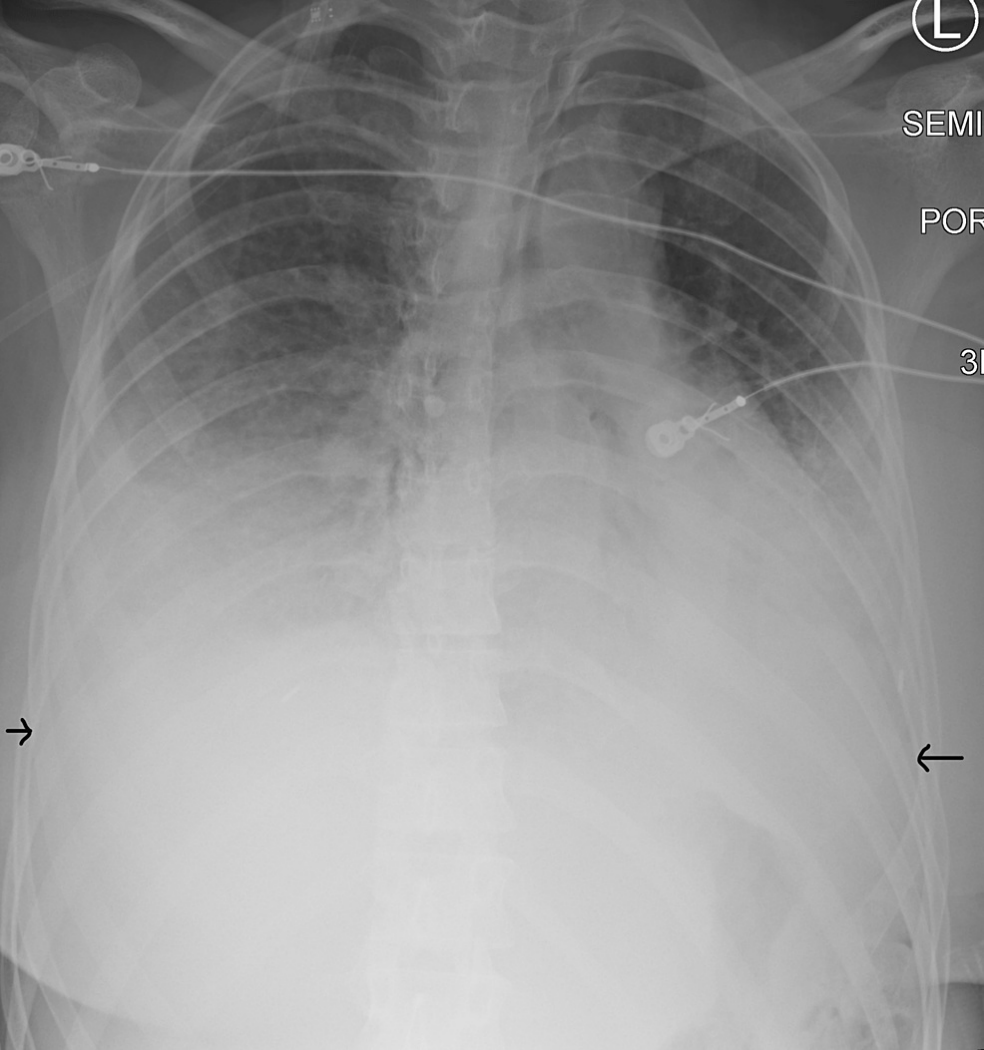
Chest radiograph on hospital day two with worsening of bibasilar hazy opacities L: left
